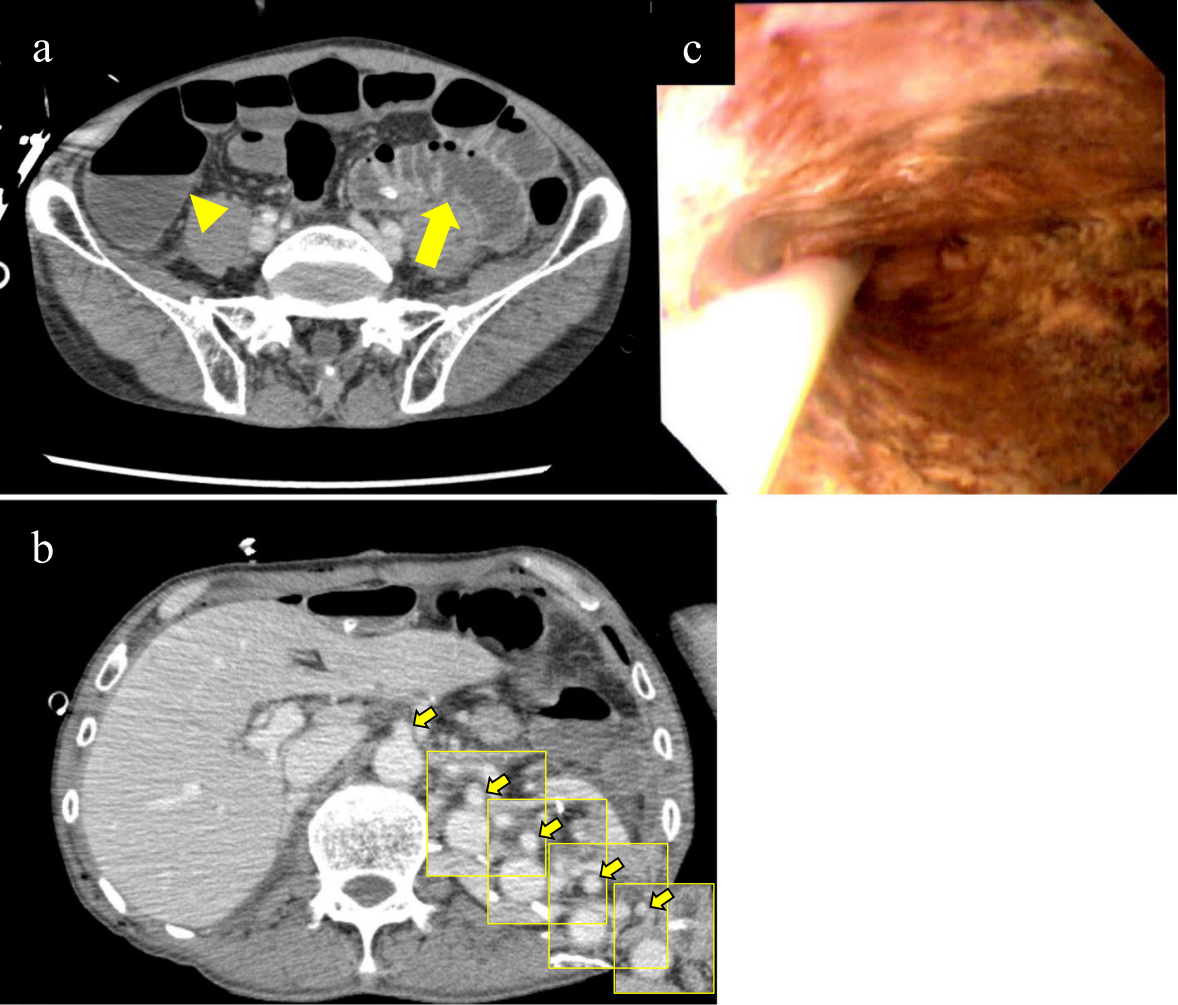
Computed tomography (CT) and endoscopy at postoperative day 2 following esophagectomy. a CT revealed dilation of the small intestine (arrow) and large intestine (arrow head). We interpreted this finding as enteritis at first. b These images show slices of the superior mesenteric artery (SMA). CT revealed that there was no thrombus in the SMA (arrow). c Upper gastrointestinal endoscopy revealed ischemic changes in the mucosa over the entire circumference of the gastric tube from the anastomotic site to the antrum
